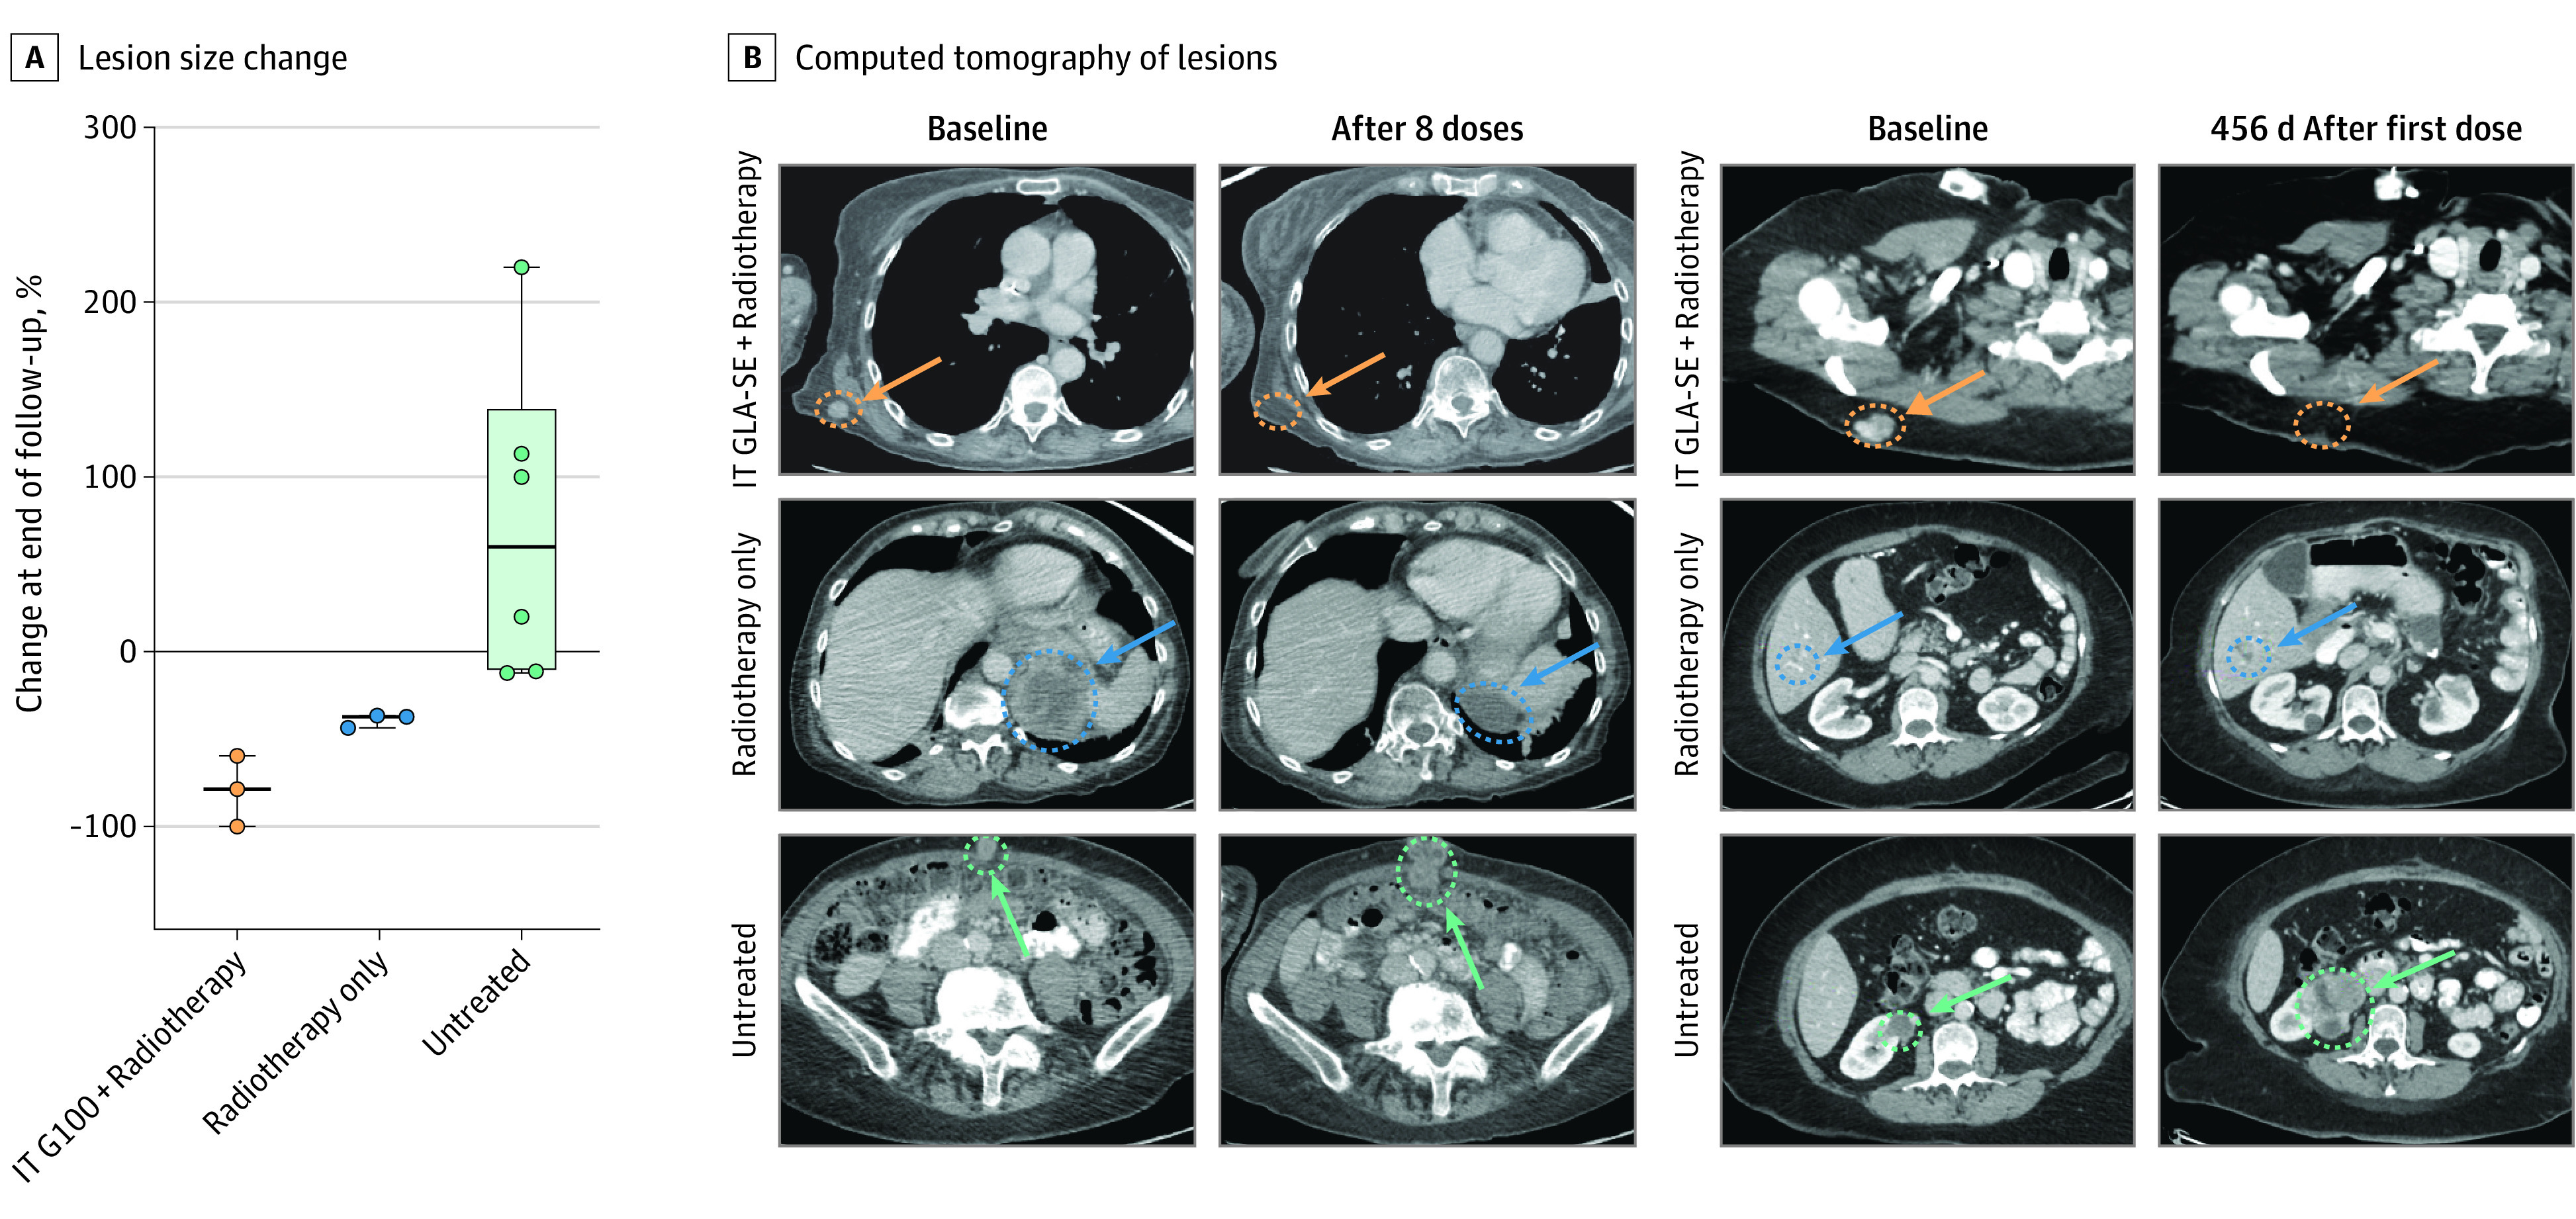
Local Control After Intratumoral Glycopyranosyl Lipid A in Stable-Emulsion Formulation (IT GLA-SE) Compared With Concomitant Lesions A, Percent change in size of lesions at end of same follow-up period for tumors receiving IT GLA-SE plus radiotherapy (orange) vs tumors receiving radiation only (blue) or no local treatment (green). B, Representative computed tomograms of lesions at baseline and at last follow-up in a patient with undifferentiated round cell sarcoma and complete regression of tumor after IT GLA-SE and patient with leiomyosarcoma −79% in size after IT GLA-SE.
